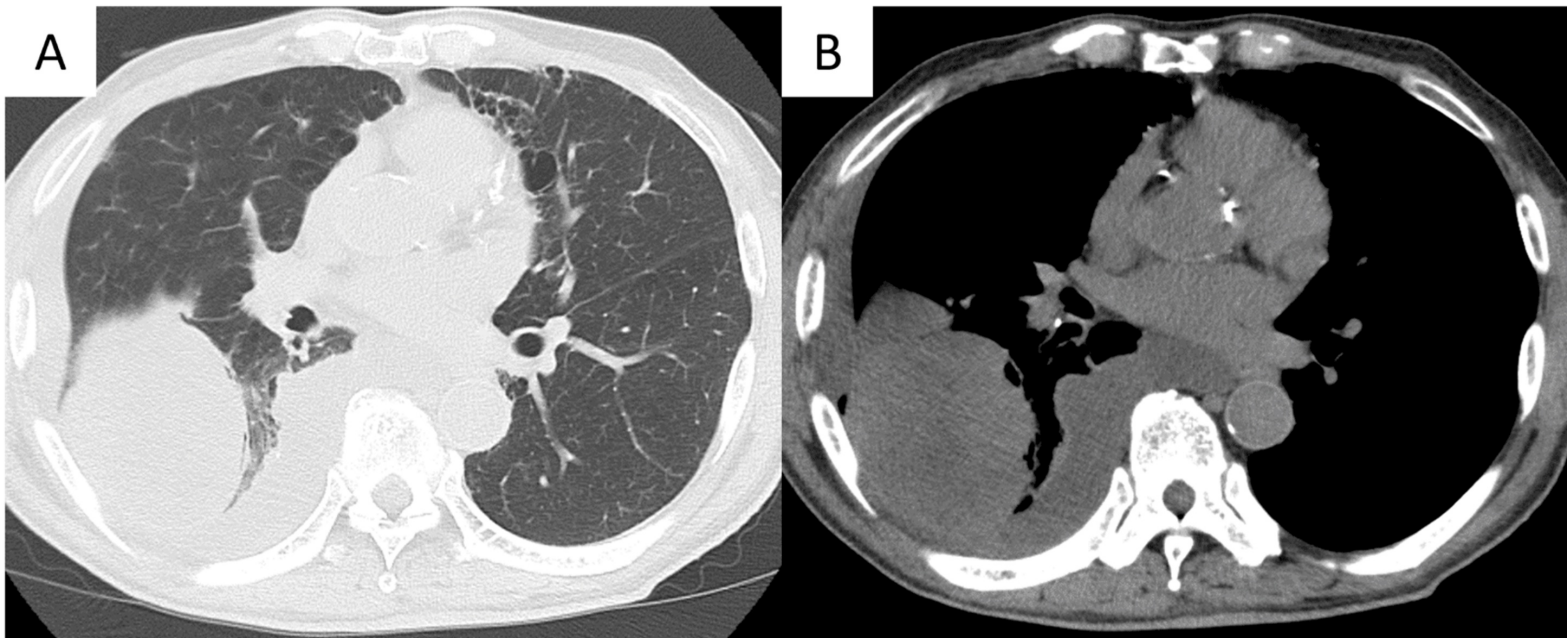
Chest computed tomography findings of chest at first admission Non-contrast chest computed tomography scan at first admission showed moderate amount of pleural effusion and a mass of 60x50x80 mm with heterogeneous internal density in the right lower lobe. A: pulmonary window, B: mediastinal window
